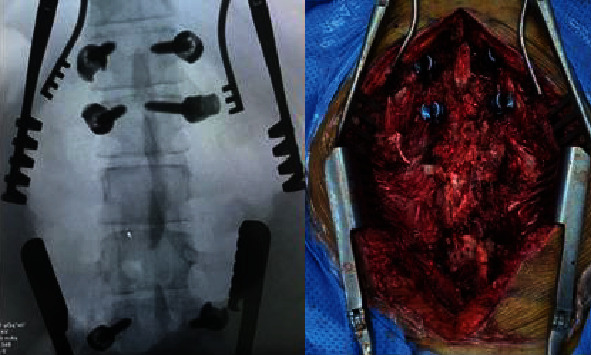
X-ray AP view and intraoperative image post-intrapedicular screw insertion and laminectomy.
